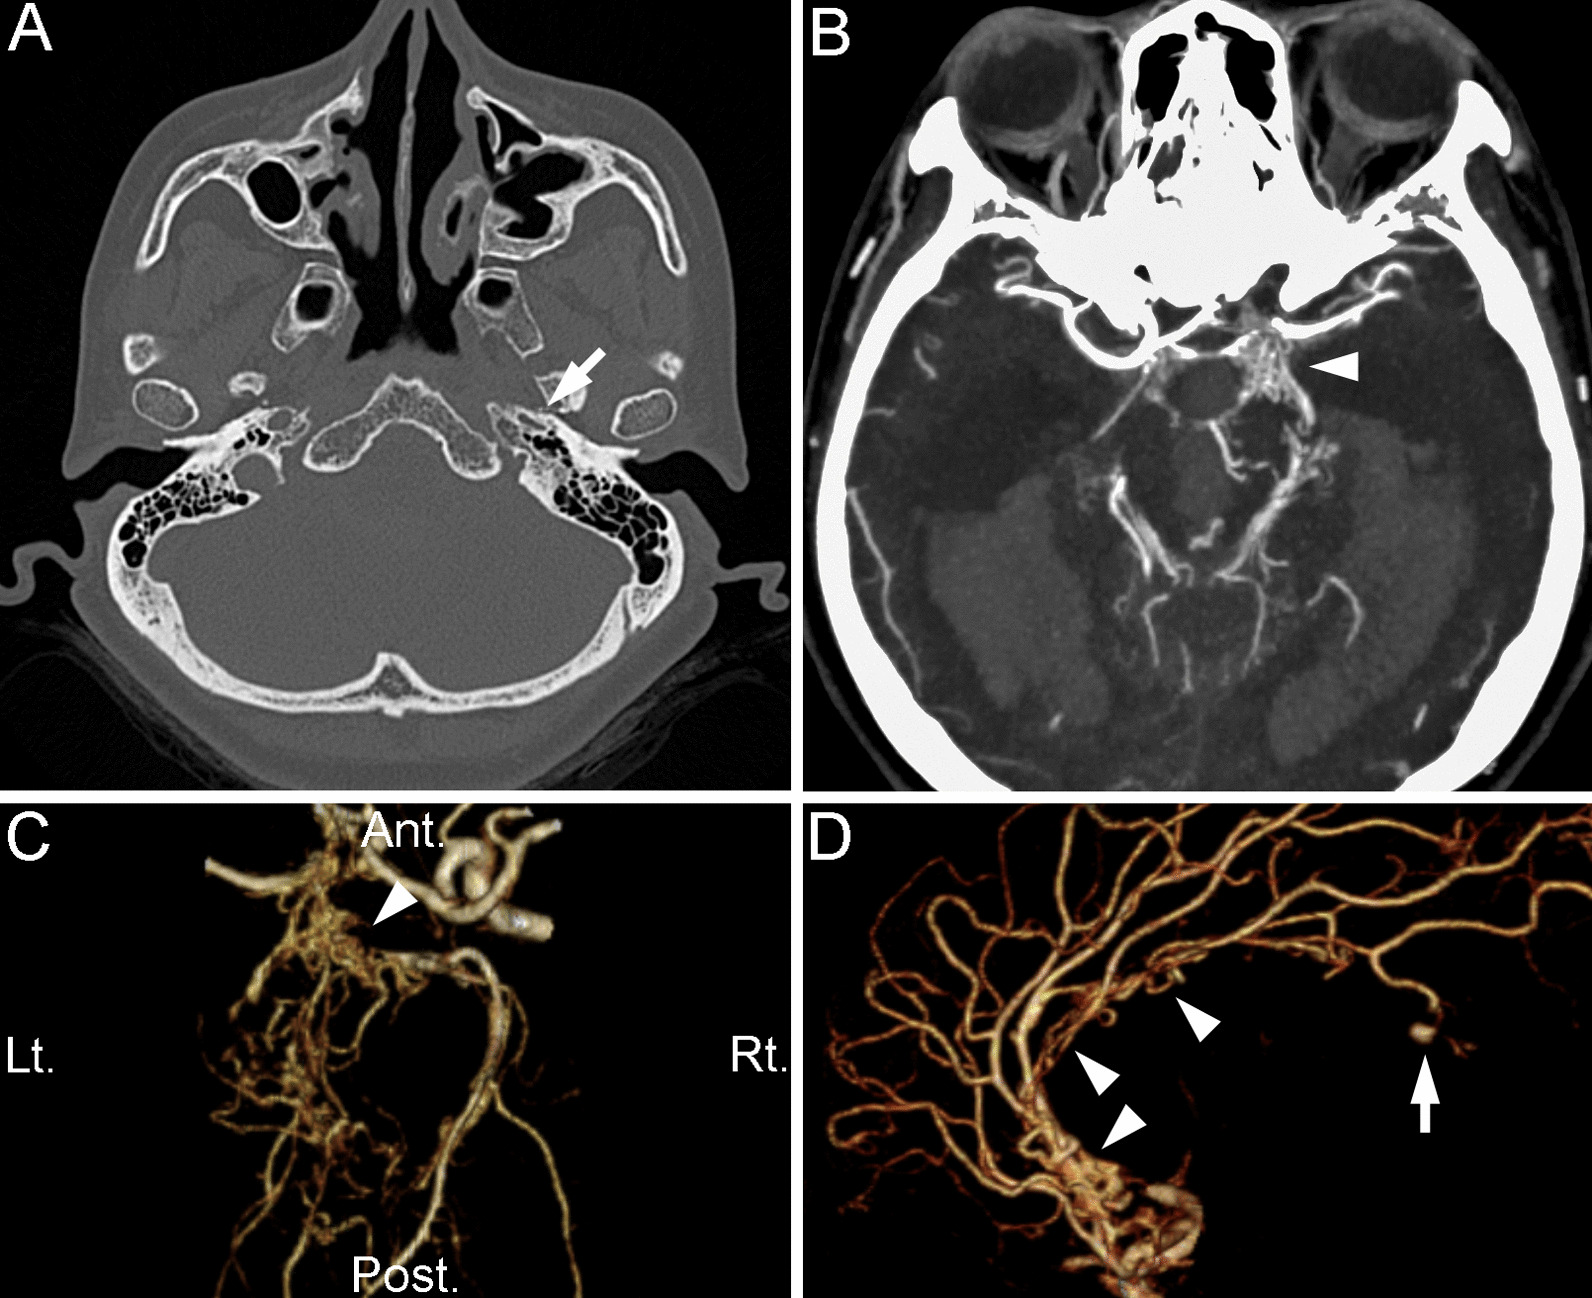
Bone window computed tomography (CT) and CT angiography (CTA). A Axial plain CT of the bone window. The arrow indicates the hypoplastic left carotid canal. B Axial CTA. The arrowhead indicates the rete mirabile of the left posterior communicating artery (PComA). C, D Three-dimensional CTA of the right–left lateral 60° view C and left–right lateral 15° view (D). C The arrowhead indicates the rete mirabile of the left PComA and the proximal segment of the posterior cerebral artery. D Arrowheads indicate the rete mirabile of the anterior cerebral artery (ACA) and the arrow indicates a distal ACA aneurysm. *Ant.* Anterior, *Lt.* left, *Post.* Posterior, *Rt.* right
